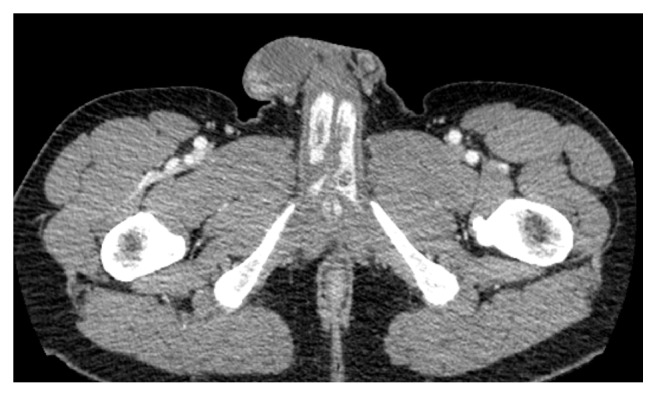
CT depicting bilateral arteriocavernous fistulae with pooling of contrast medium in the corpora.
